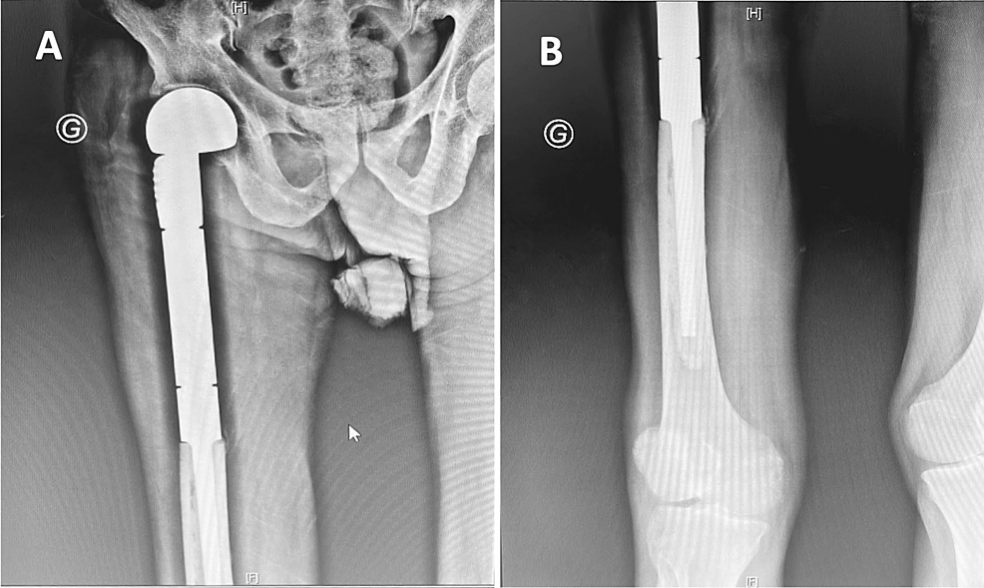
The postoperative X-ray shows (A) the proximal femur prosthesis and (B) its insertion into the distal medullary canal.
